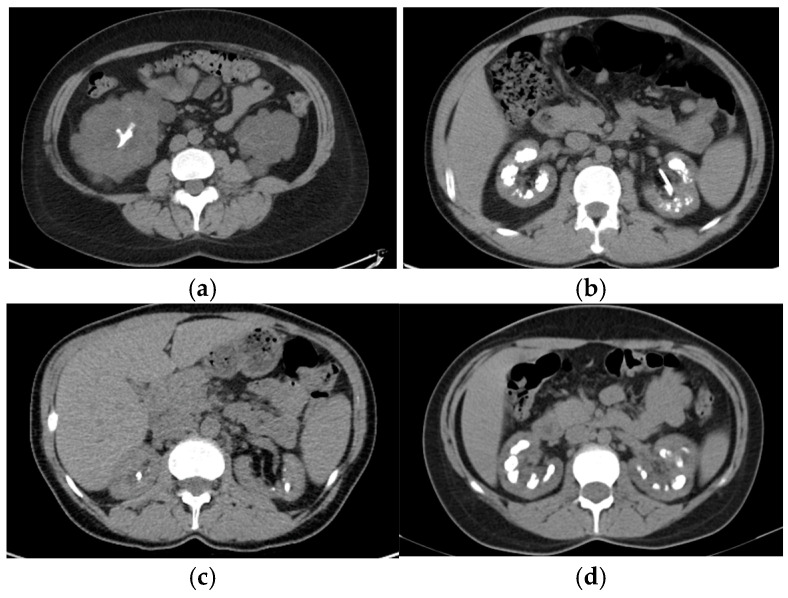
Renal imaging from 4 patients. (a) CT scan of the 43-year-old patient with cystinuria and autosomal dominant polycystic kidney disease, transversal section: staghorn calculus in the right kidney; (b) CT scan of the 39-year-old patient with hereditary dRTA, transversal section: non-obstructive multiple bilateral kidney stones and nephrocalcinosis, left ureteral JJ stent; (c) CT scan of the 42-year-old patient with PH1, transversal section: non-obstructive bilateral kidney stones and intraparenchymatous calcifications; (d) CT scan of the 32-year-old patient with Bartter syndrome type 2, transversal section: bilateral medullary nephrocalcinosis.
